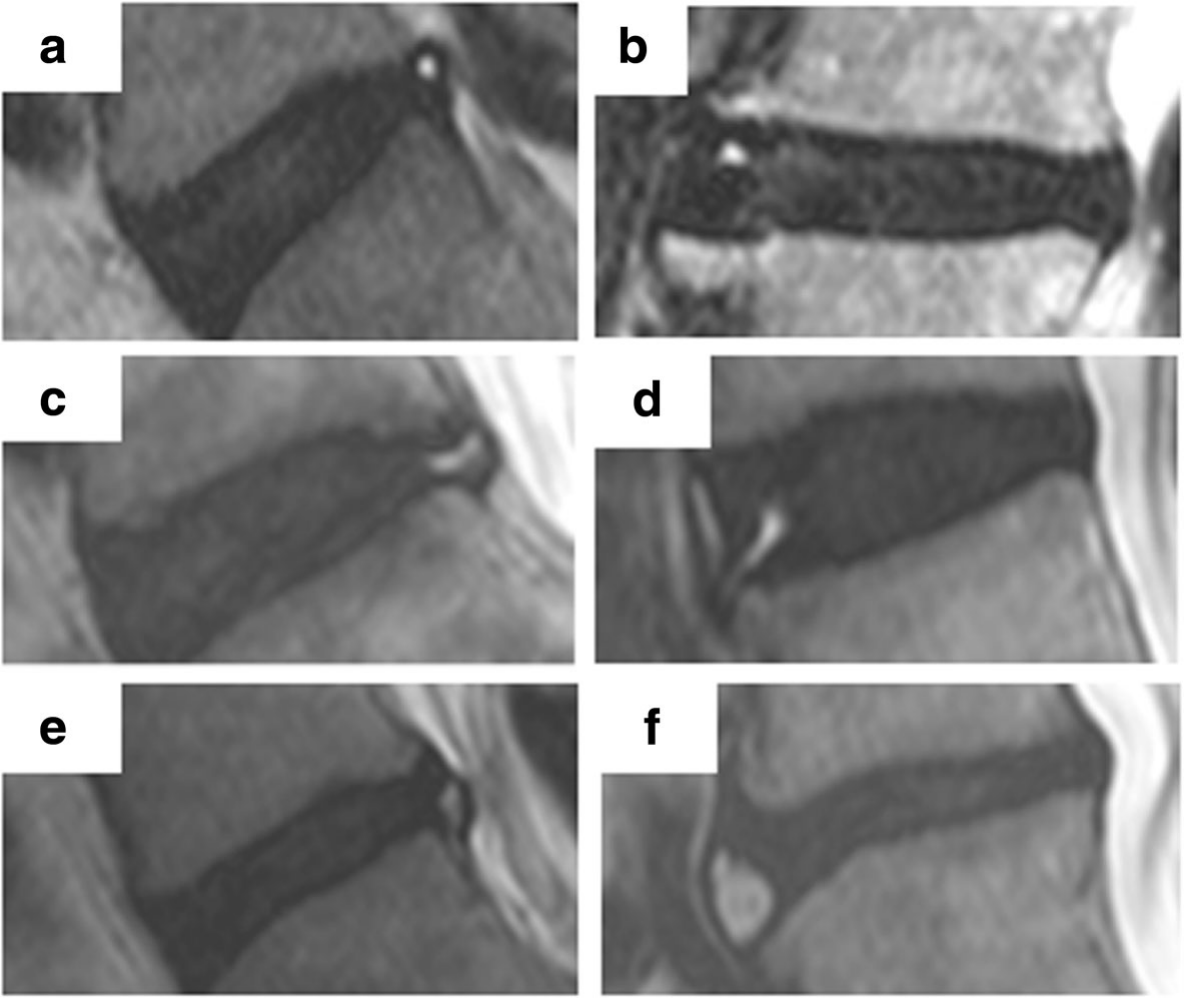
Novel classification of HIZ on T2-weighted MRI by Teraguchi et al. a Posterior round type. b Anterior round type. c Posterior fissure type. d Anterior rim type. e Posterior vertical type. f Anterior enlarge type
